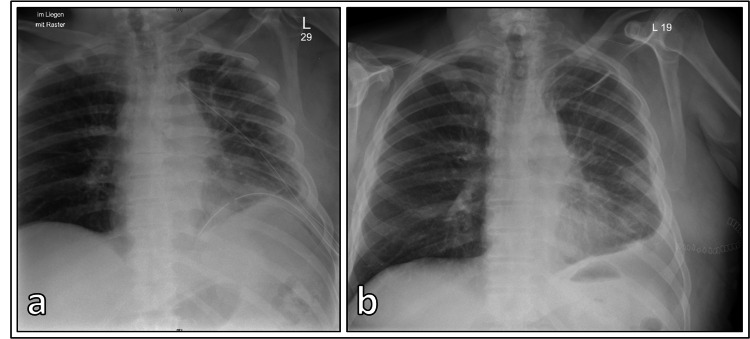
X-ray images after the surgery a. Direct postoperative X-ray after repair and b. after the removal of the chest drains.
